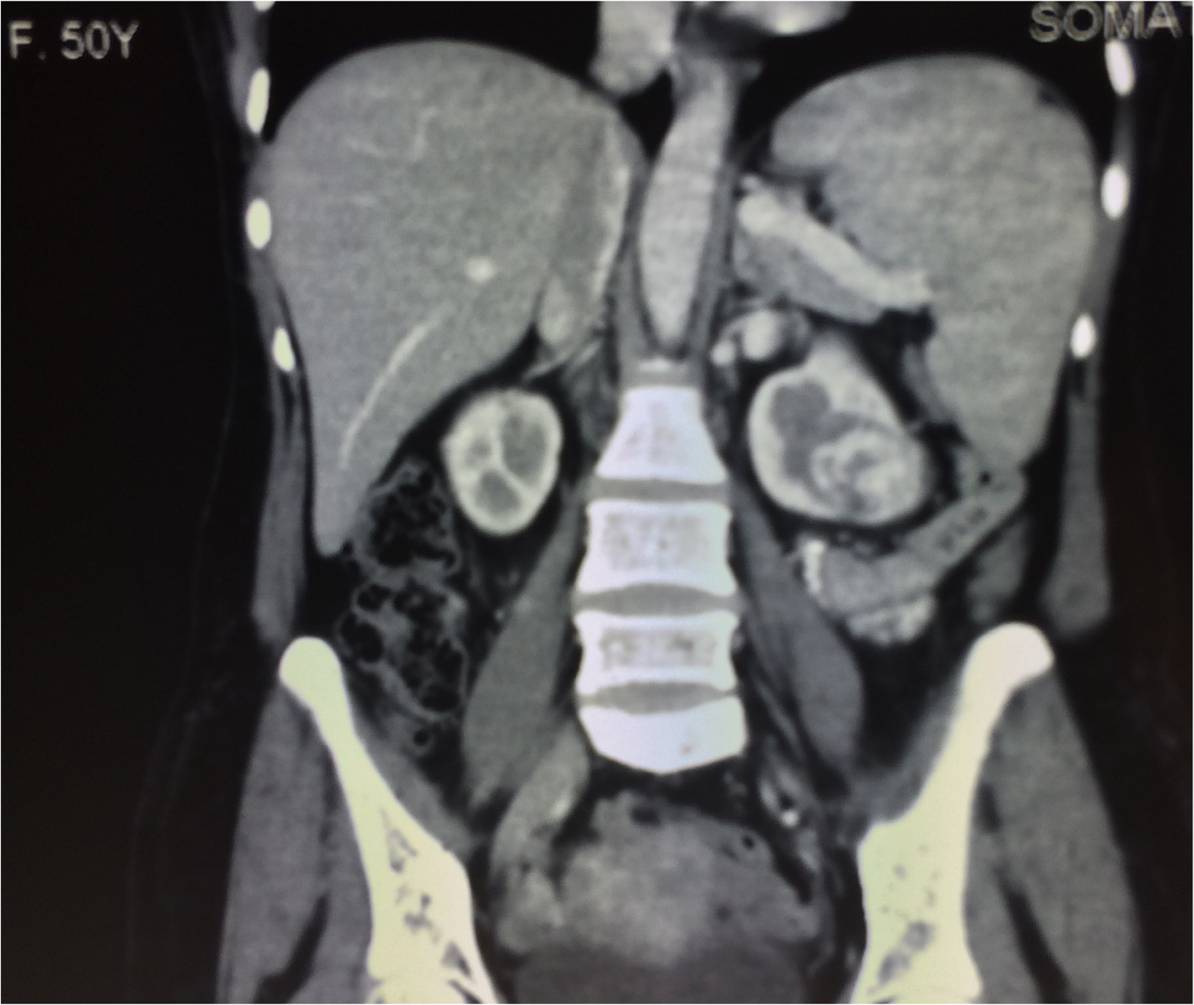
MRI (coronal section) showing renal mass reaching to pelvicalyceal system
